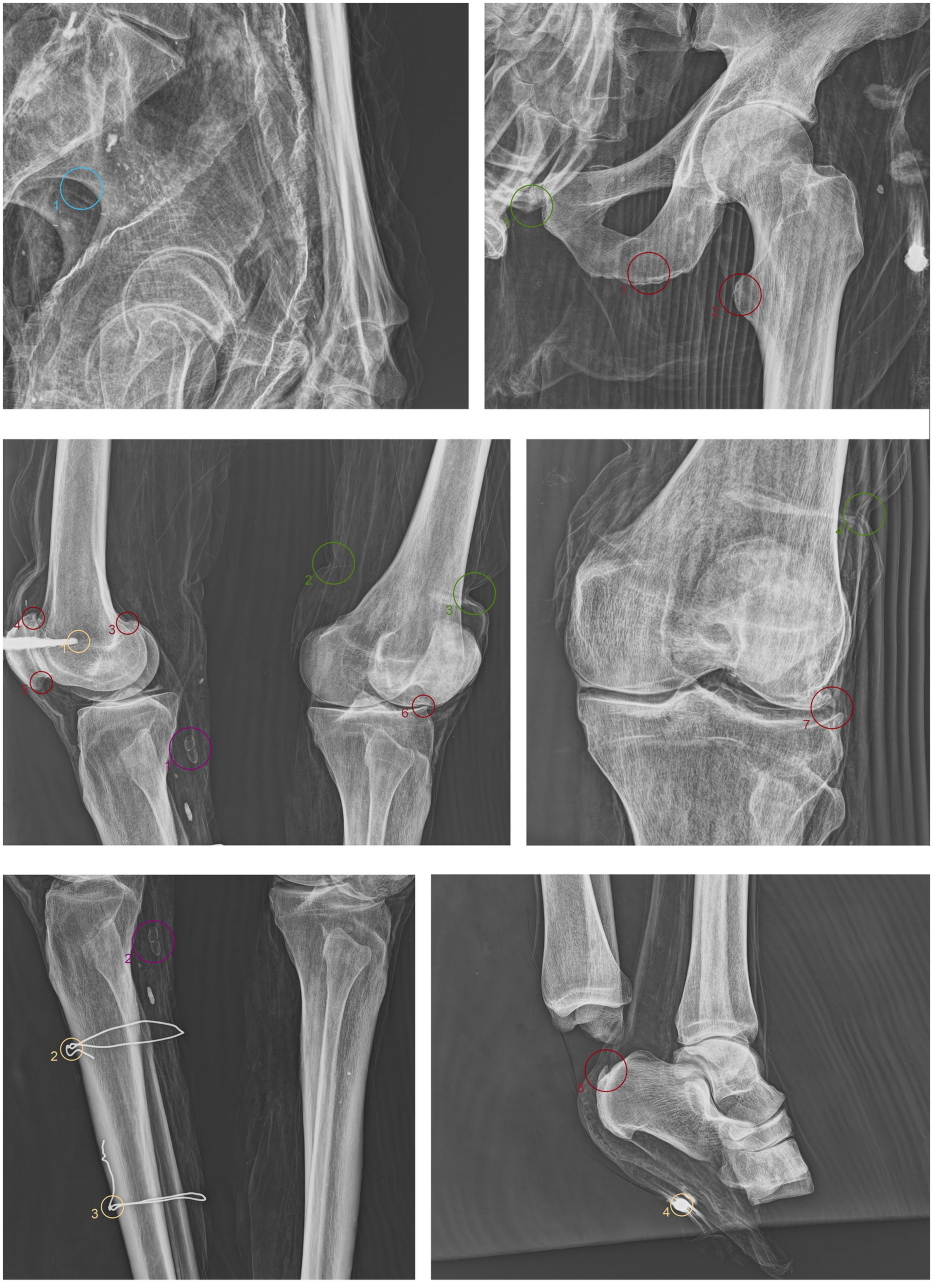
Anteroposterior and lateral radiographic acquisitions of the pelvis and lower limb of individual ID2. Green highlights indicate areas of interest for taphonomic study, yellow highlights indicate areas relevant to the assessment of restorations predating this study, violet is used for entomological analysis, blue for anthropological aspects, and red for paleopathological evidence.
